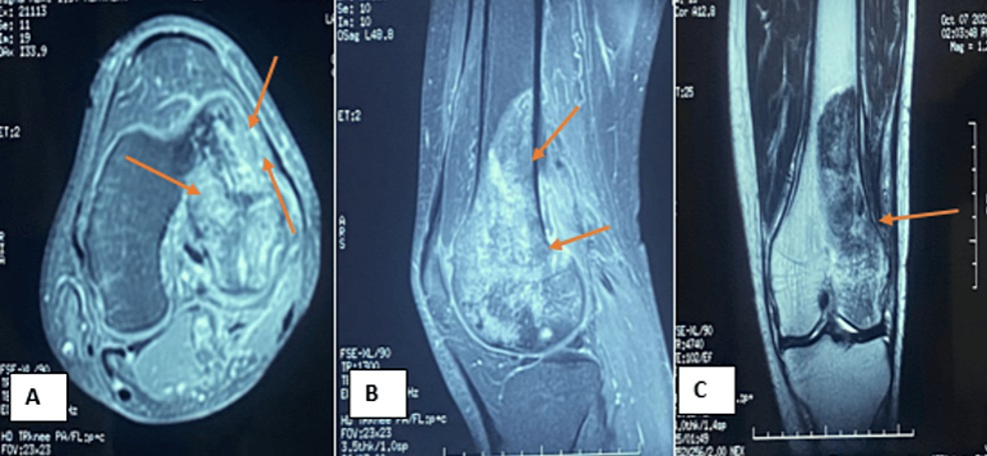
MRI of the patient after chemotherapy. (A) MRI coronal T2-Weighted image showing a osteoblastic lesion of the lateral condyle. (B) MRI sagittal T2-weighted image reveals the extension of the tumor to the femoral biceps muscle. (C) MRI axial T1-weighted image reveals the dimensions of the tumor.
